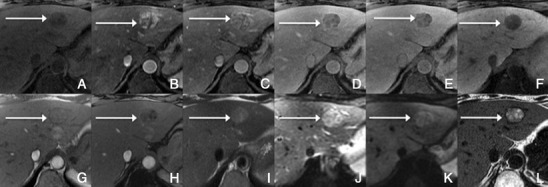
Fatty HCC in a 45-year-old man with chronic liver disease. a Pre-contrast MRI, b, c arterial phase, d 3 min, f 4 min, f 8 min, g IP, h OP, i SSFSE, j b0, k b500, l T1-weighted PDFF. The *arrows* point to a rounded focal lesion, with arterial enhancement, PV washout and hypointensity in the HCP. It also shows heterogeneous loss of signal in OP (fat), expressed likewise in the fat fraction map as bright areas in the lesion
